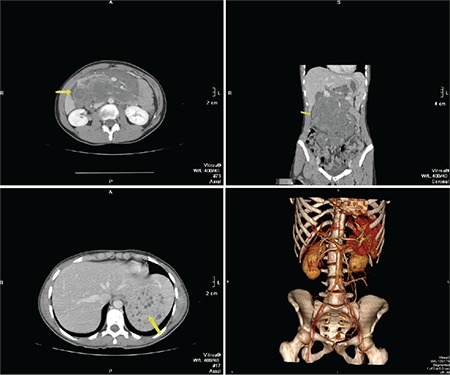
Multislice computed tomography showed a large retroperitoneal tumor.
